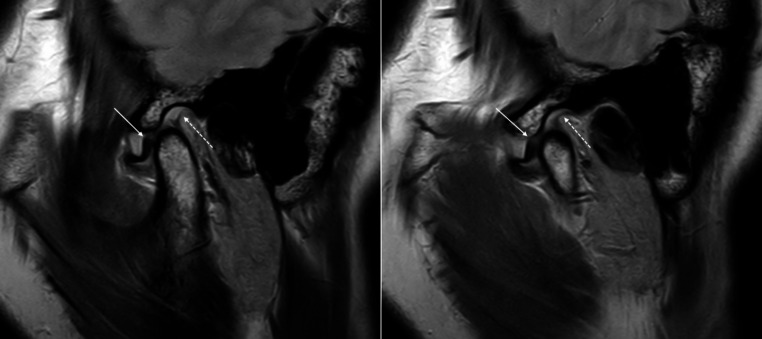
Two consecutive modified-sagittal proton density-weighted MR images with open mouth position, demonstrating retrodiscal layer rupture of the right temporomandibular joint (patient #14). Note the separation between the posterior band of the disc (arrow) and the retrodiscal layer (dashed arrow)
